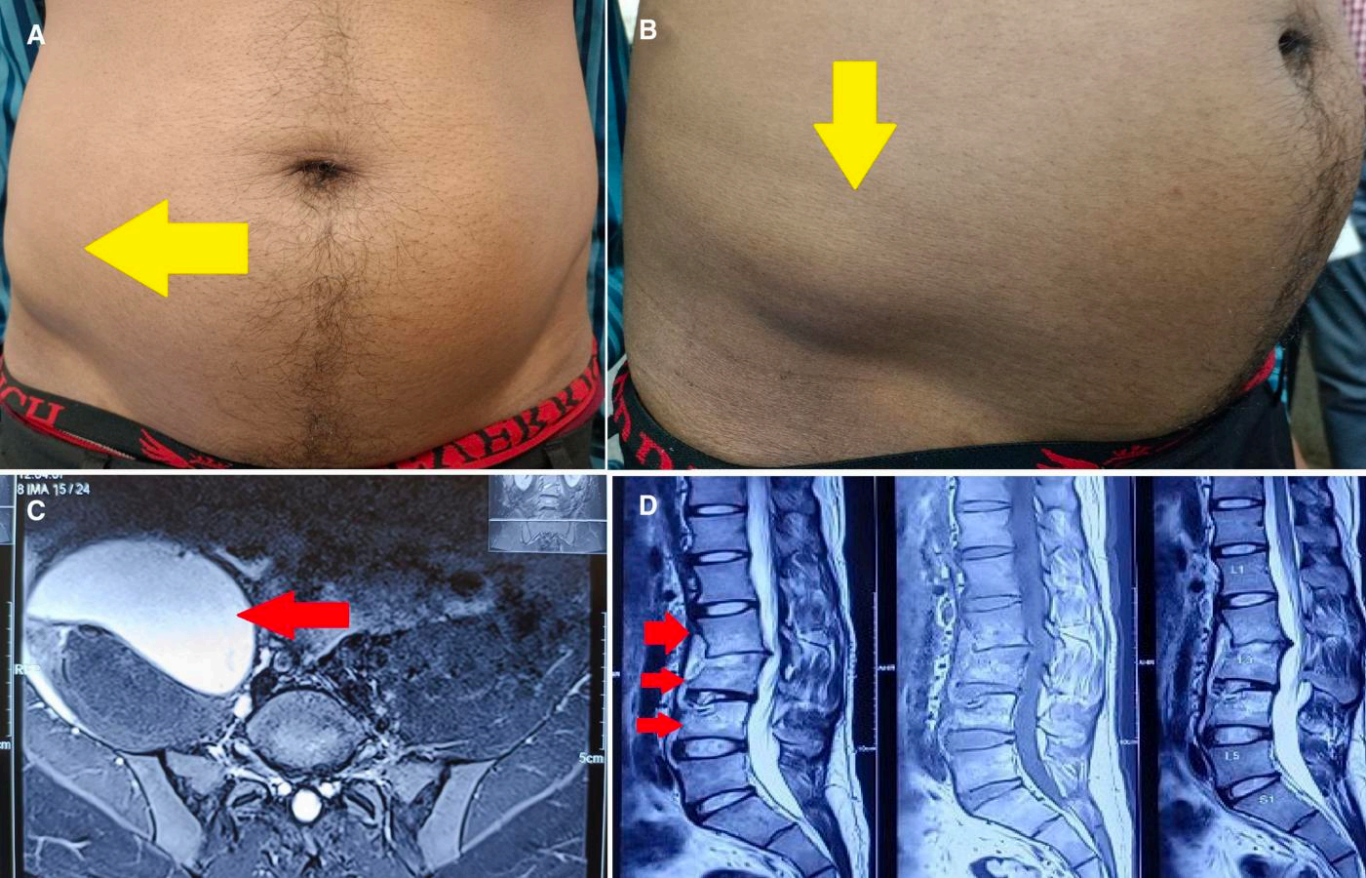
Psoas abscess in spinal tuberculosis. (A and B) Clinical image showing fullness of right lumbar and right iliac area (yellow arrow in front view and right lateral oblique view). (C) Axial magnetic resonance imaging (MRI) showing right large psoas abscess (red arrow). (D) Sagittal MRI showing tubercular involvement of multiple lumbar vertebras (L2, L3, L4) (red arrows).
